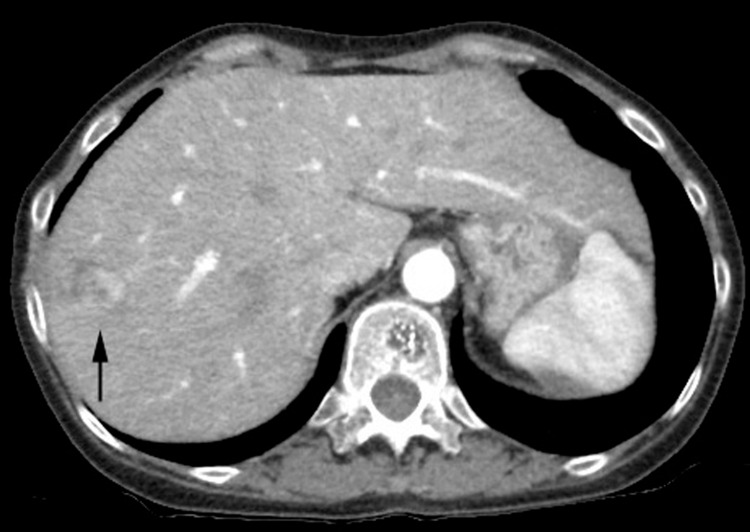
Abdominal CT. CT scan showing a heterogeneously enhancing hepatic tumor (arrow) at the arterial phase. CT: computed tomography
